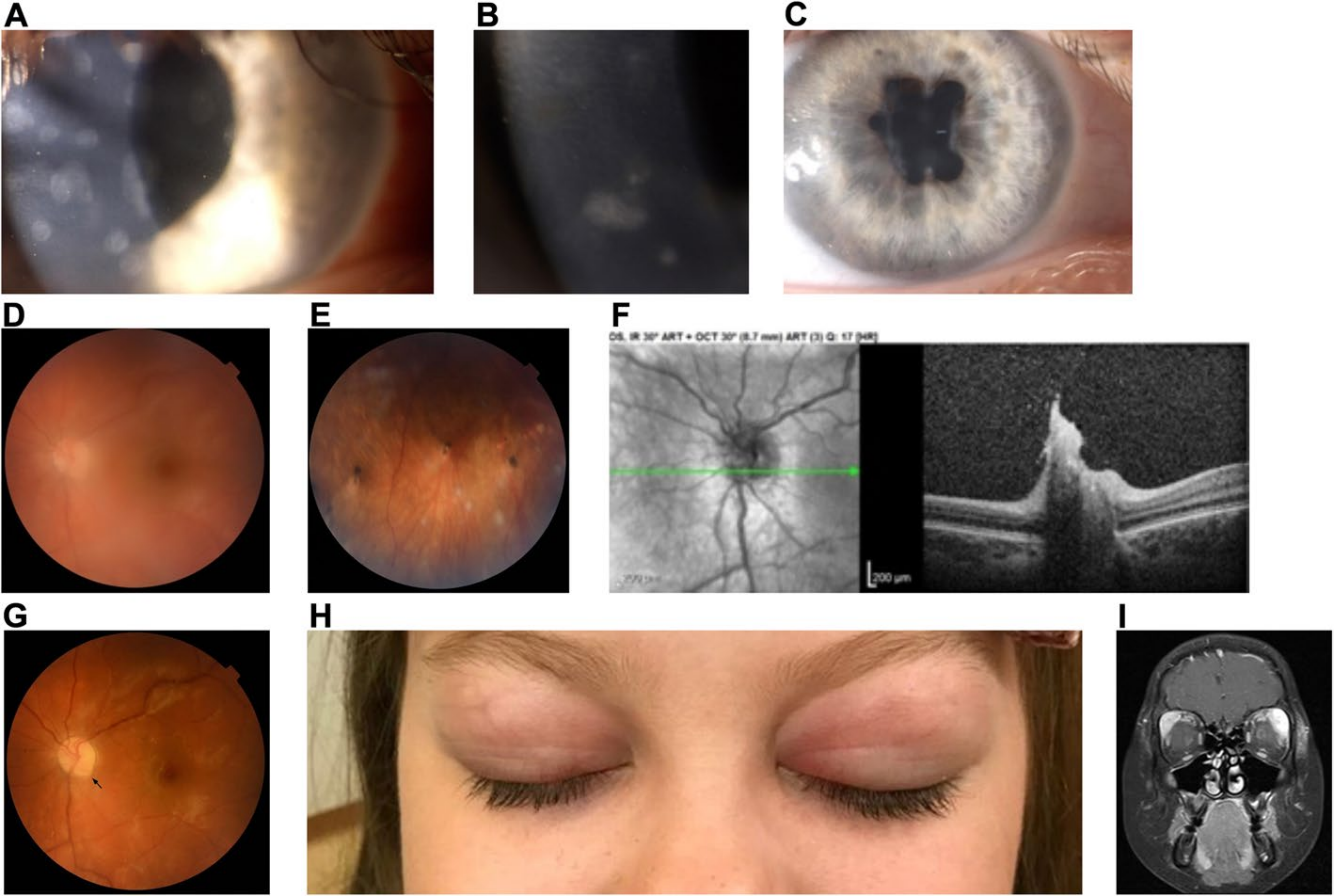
A pediatric female patient with suspected Blau Syndrome with de novo NOD2 mutation was followed for 10 years (2–12 y.o.). During the course of the disease, bilateral corneal old and new active subepithelial nummular infiltrates were noted and visualized here on color photographs of the right eye (A) and (B). The patient also developed bilateral posterior synechiae visualized here on color photograph of the right eye (C). Her fundus examination revealed bilateral vitritis and multifocal choroiditis with active and inactive peripheral lesions visualized on the left eye fundus color photography (D, E). Left eye optic disc granuloma, which appeared during relapse on adalimumab, was documented on the OCT (F) and later on the fundus color photography (G) after vitritis resolution following treatment with infliximab. Several years later, during another relapse initiated by switching to anakinra treatment, the patient developed Mikulicz’s syndrome with enlargement of bilateral lacrimal glands visualized on (H) color photography and on (I) brain MRI, coronal plane. The disease is now controlled with JAK-1 inhibitor baricitinib. Black arrow: optic disc granuloma
